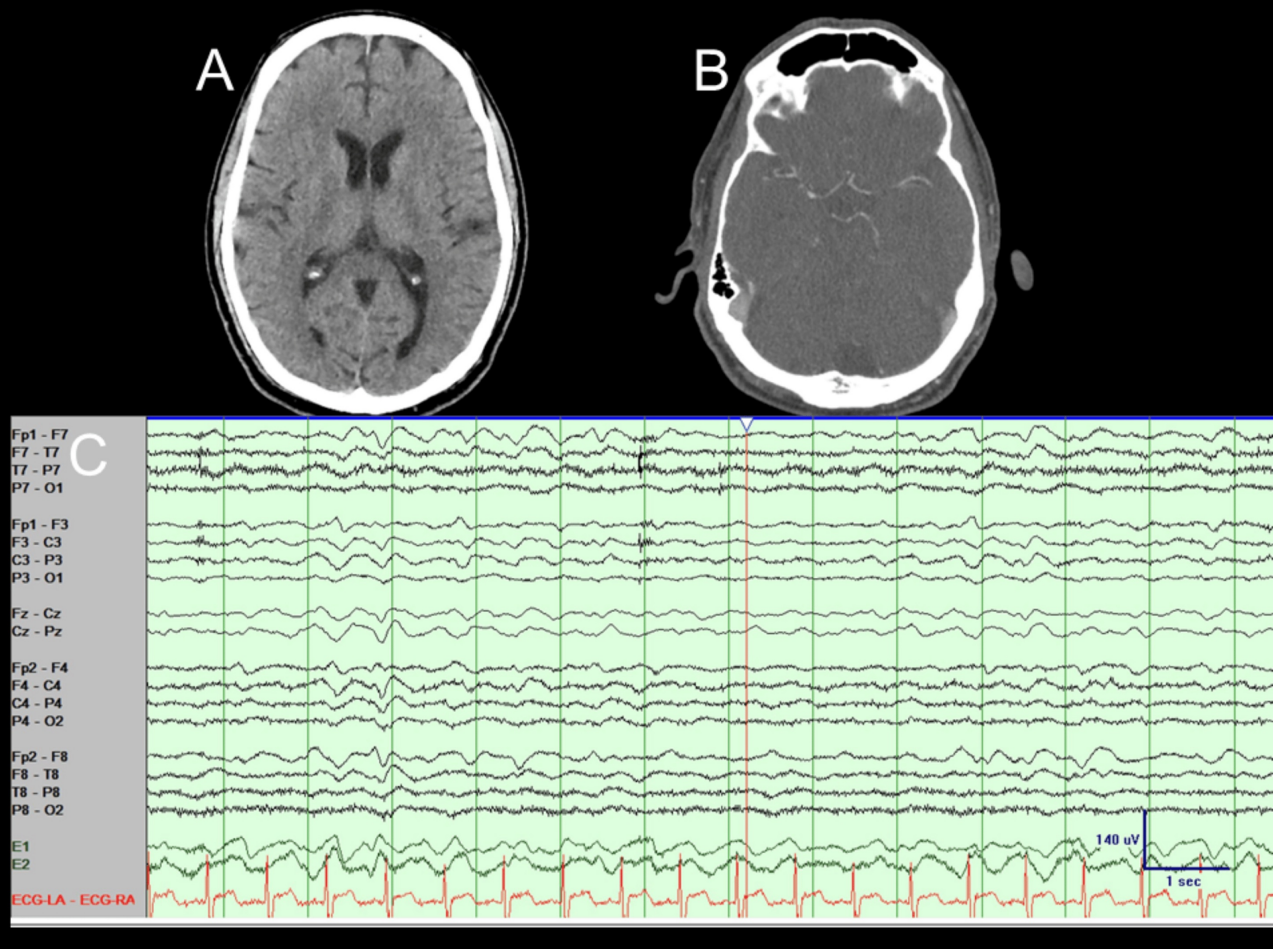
Initial diagnostic workup including CT and CTA of the head and EEG CT and CTA of the head were negative (A and B). The EEG (C) showed diffuse slowing background lacking normal awaking or sleep organization, without discernible posterior dominant rhythm and non-epileptiform abnormalities or electro-clinical seizures, which is suggestive of moderate diffuse encephalopathy. CTA: CT angiogram; EEG: electroencephalogram
